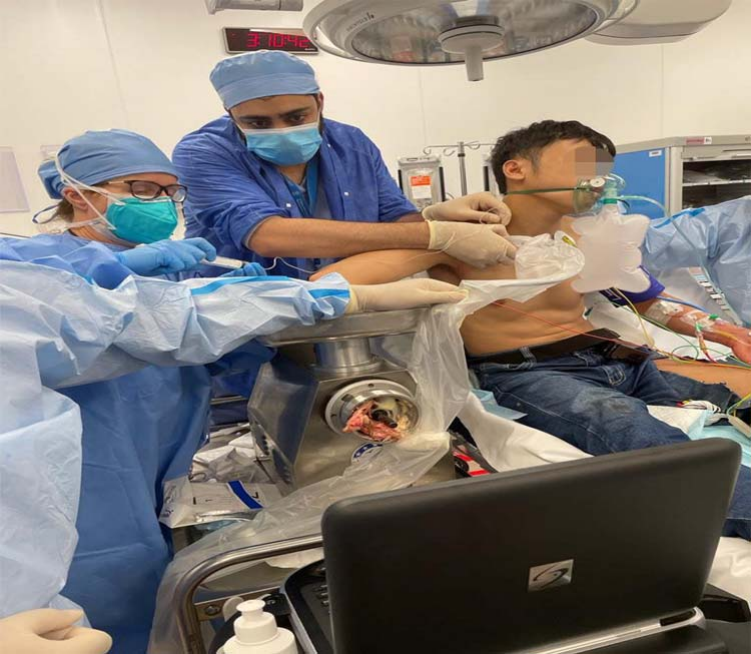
The patient is in a feasible position with ultrasound guided infraclavicular plexus block for extraction of the arm in place.
